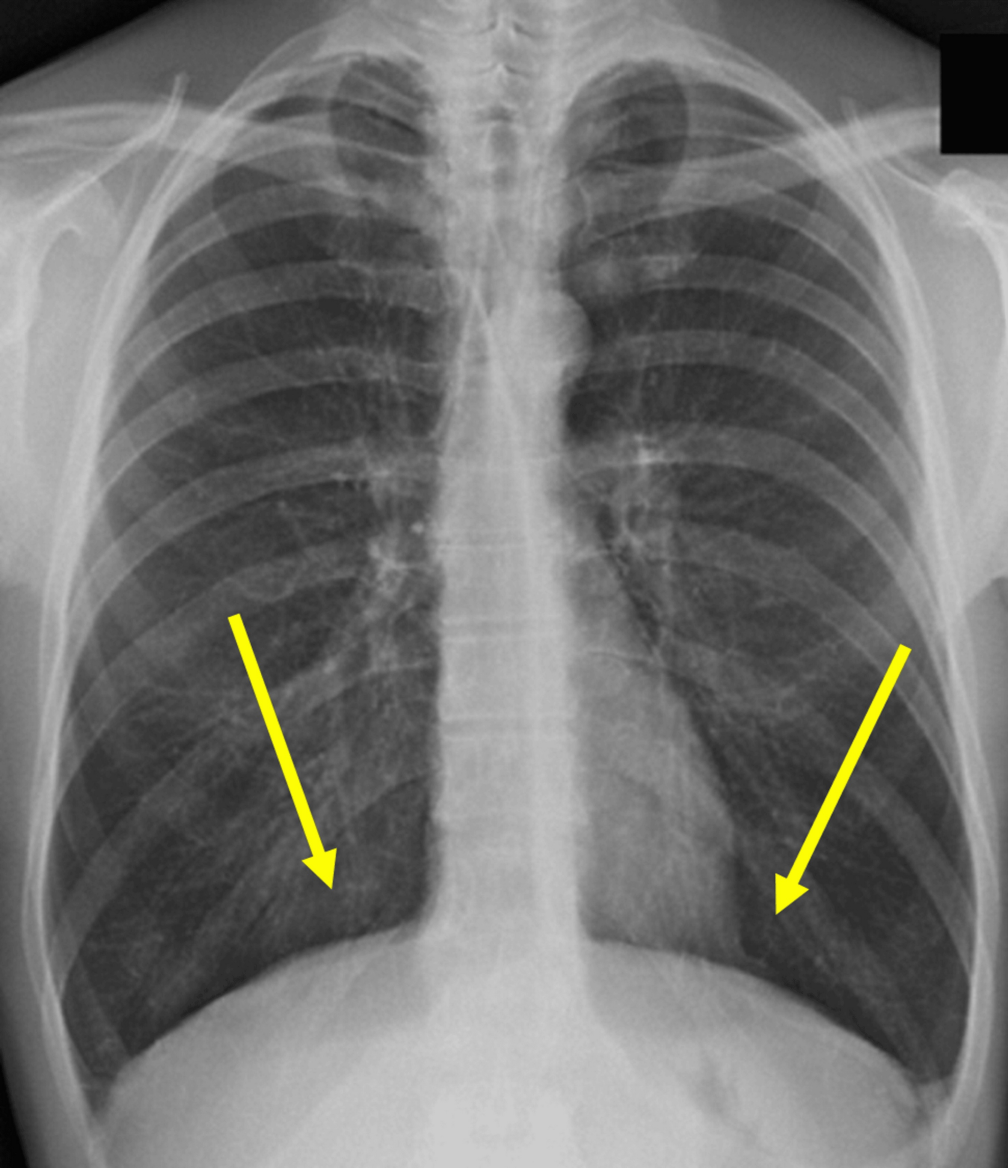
Chest X-ray of a COPD patient. The "rounded" morphology (arrows) of the diaphragm is noted, which is in an inspiratory posture, with shortened fibers COPD: chronic obstructive pulmonary disease Image Credit: Bruno Bordoni
